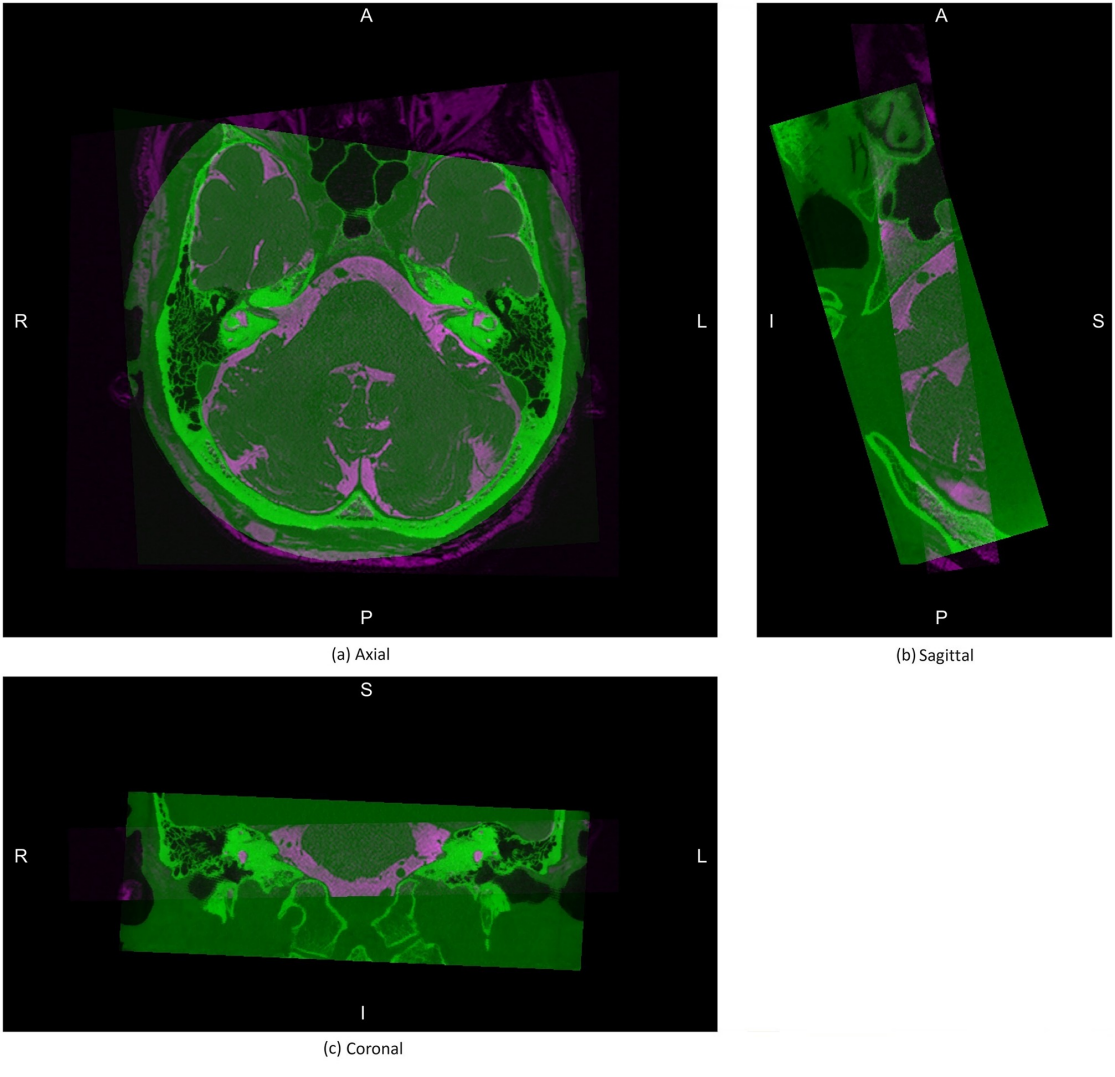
Overlap between the mid slices of the CT and MR images in the proposed coordinate system. A, P, S, I, R, and L indicate anterior, posterior, superior, inferior, right, and left directions respectively.
